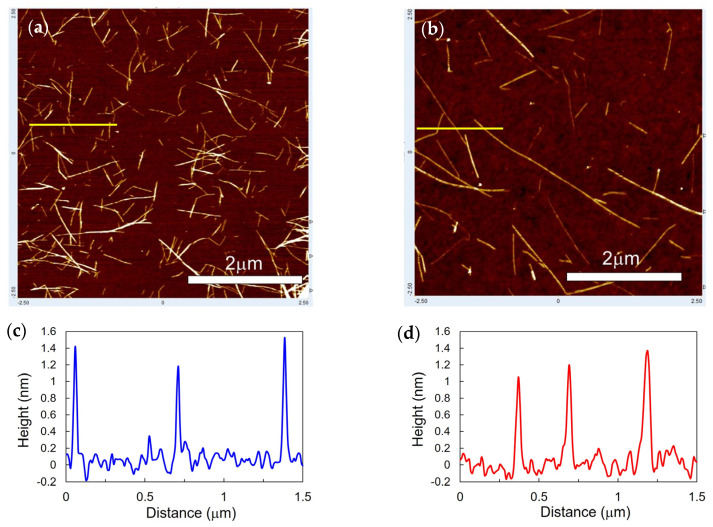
(a,b) AFM images SWCNTs on SiO_2_/Si, and (c,d) the height profiles for yellow lines specified in the images. SWCNTs were dispersed by using (a,c) ultrasonic homogenization and (b,d) HCD method.
